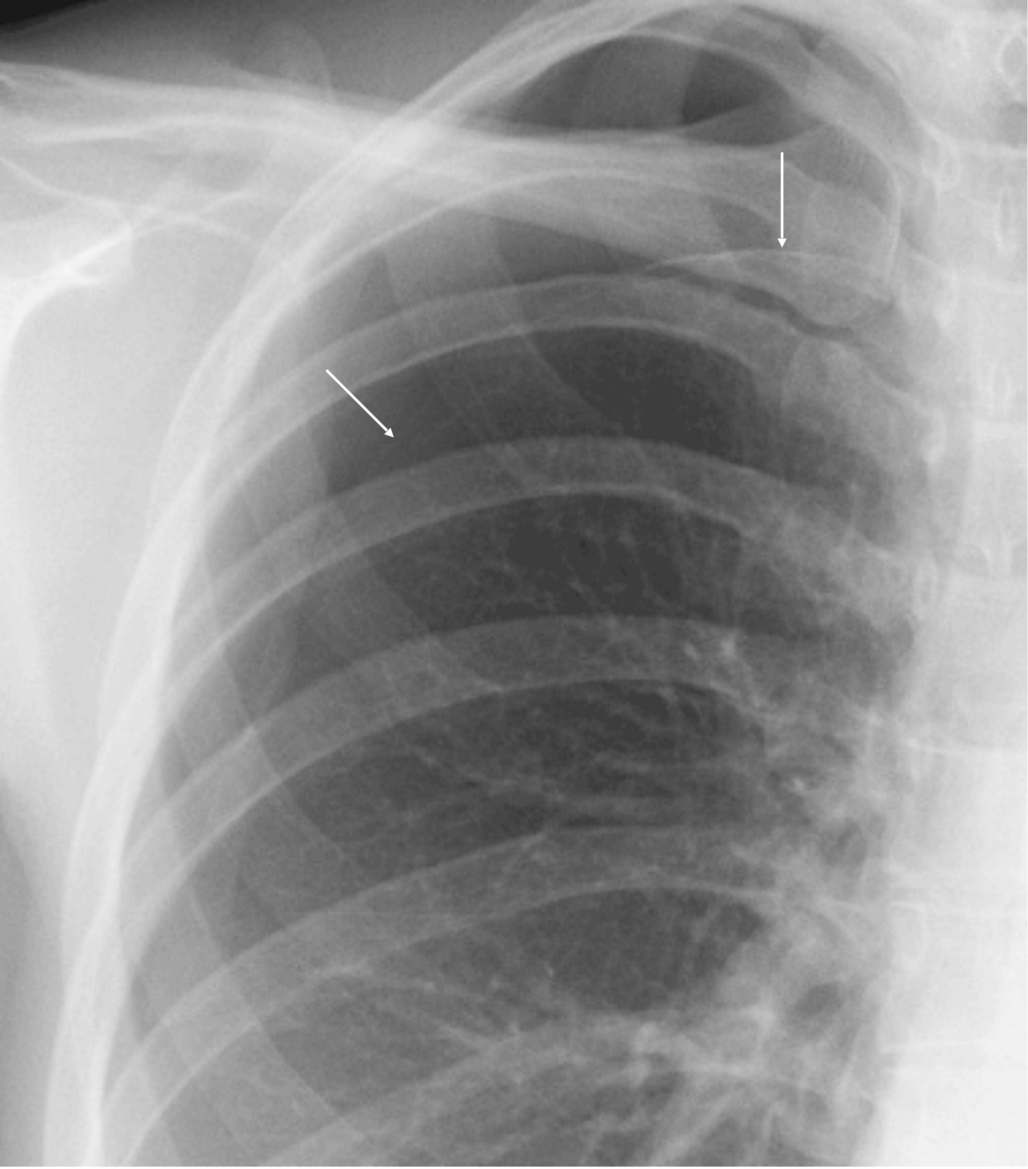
Chest radiograph showed mild collapse of the right lung.
